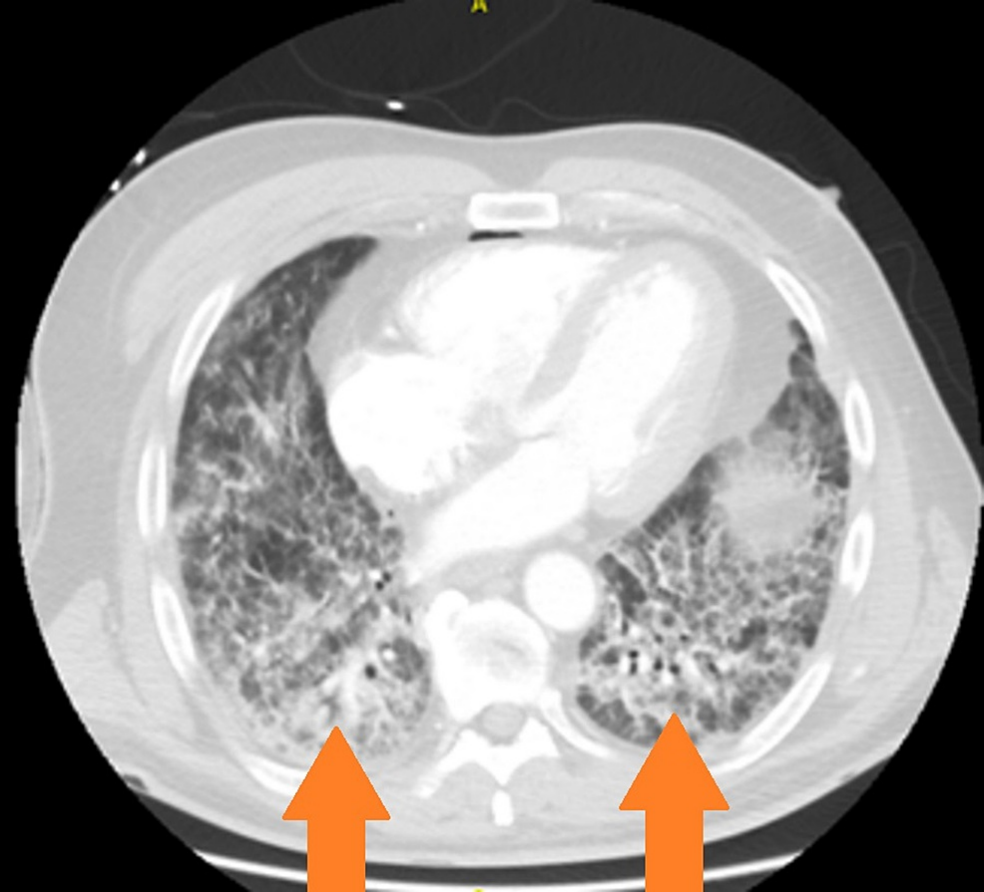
Initial chest CT scan showing dense bilateral basilar reticulations highlighted by the arrows
